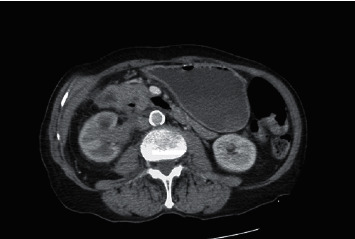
Axial view of the CT scan of the abdomen and pelvis showing a mass in the right renal pelvis.
